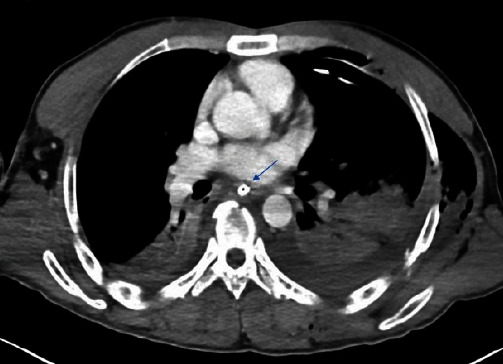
Chest CT scan showing orogastric tube (arrow).
